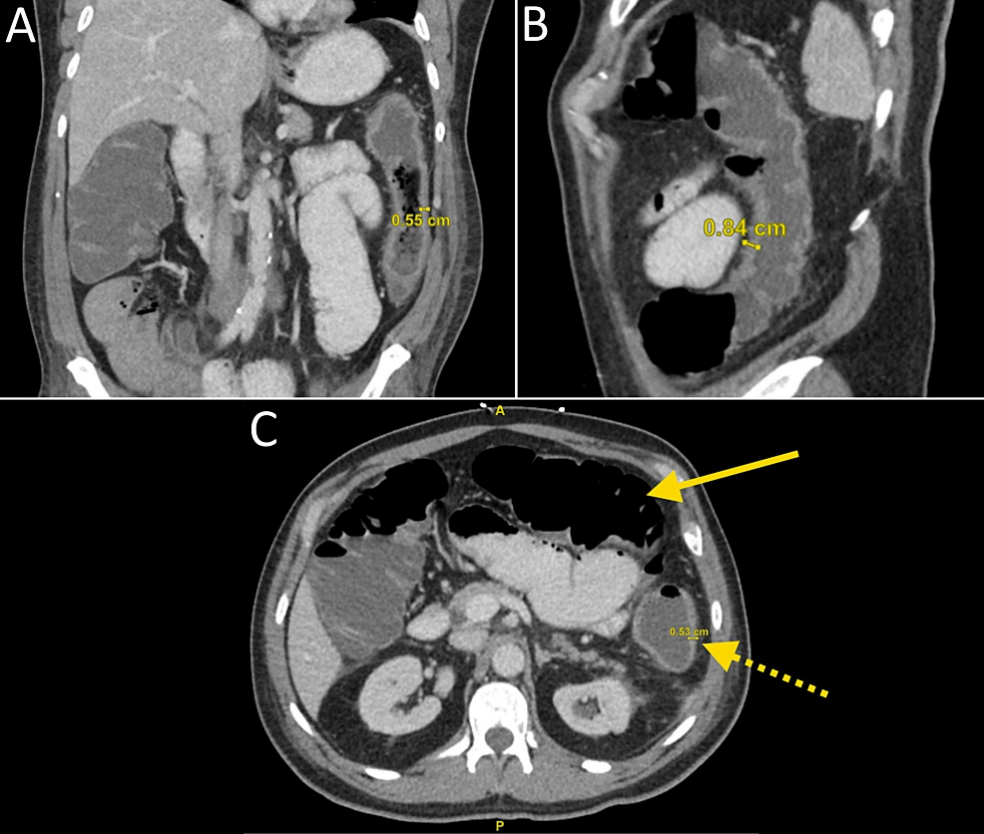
A CT scan of the patient's abdomen and pelvis with contrast showing: (A) coronal view of the descending colon with a wall thickness measuring 5.5 mm, (B) sagittal view of the descending colon with a wall thickness measuring 8.4 mm, (C) transverse view of the descending colon with a wall thickness measuring 5.3 mm as indicated by a dotted arrow and dilation of the small bowel as indicated by a solid arrow CT: computed tomography
